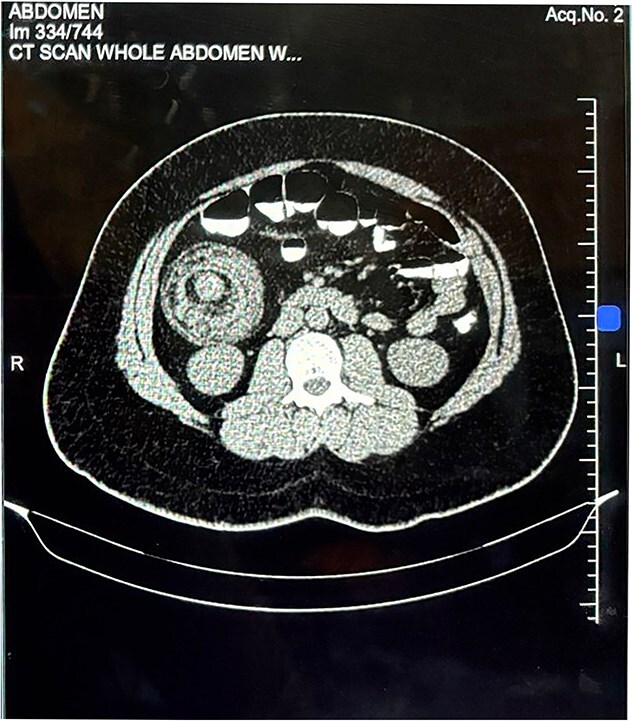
Axial contrast-enhanced computed tomography of the abdomen. Axial section demonstrating the characteristic "target sign" with a heterogeneously enhancing intraluminal mass at the lead point of the intussusception.
